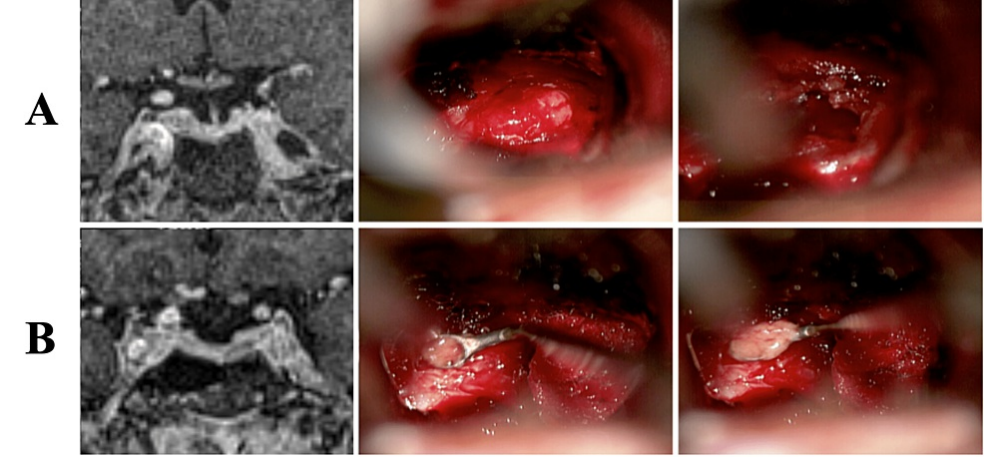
MRI from the navigation system and intraoperative images of patients with double pituitary adenomas (A) Right side. (B) Left side MRI: magnetic resonance imaging
